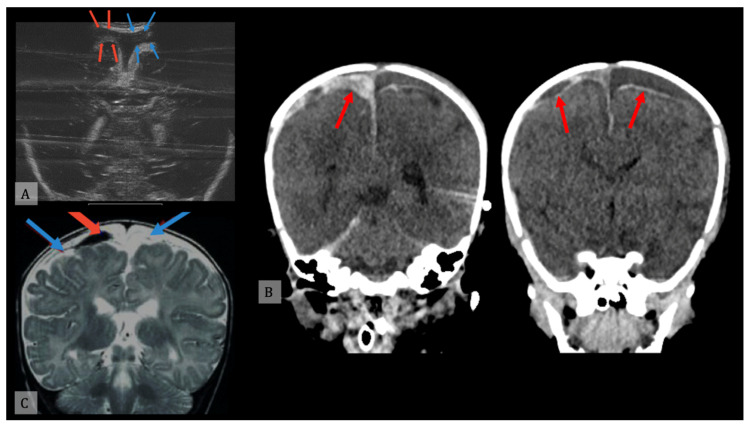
(A) Ultrasound examination of subdural (red arrows) and subarachnoid (blue arrows) hemorrhage. (B) Head computed tomography of subdural hemorrhage (red arrows). (C) Head magnetic resonance imaging of subdural (red arrow) and subarachnoid (blue arrows) hemorrhage.
